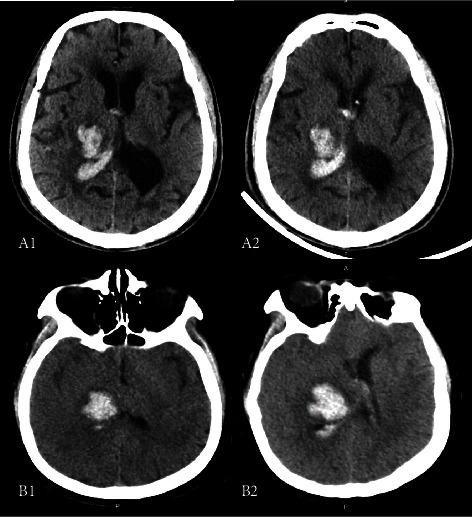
Thalamic hemorrhage with the posterior limb of internal capsule involvement. Patient A is a 71-year-old male with right thalamic hemorrhage, the baseline CT (A1) scan was performed 1.5 hours after symptom onset, and the follow-up CT (A2) showed that hemorrhage had not expanded. Patient B is a 55-year-old woman with right thalamic hemorrhage, and the follow-up CT (B2) showed hemorrhage increased by 3.66 ml than the initial volume (B1).
